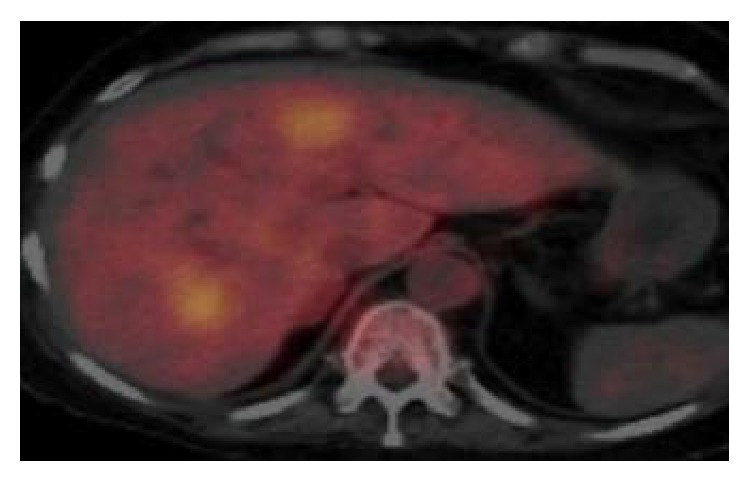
Liver metastasis (PET/CT).
